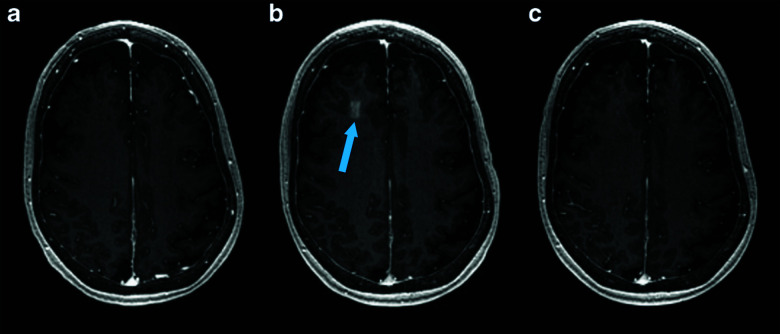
MRI images from baseline (a), immediately following HIFU (b), and 24 hours after HIFU (c), demonstrating opening and closure of BBB. Extravasation and pooling of contrast within the insonated portion of the frontal lobe is visible immediately following HIFU administration (b, blue arrow) demonstrating opening of the BBB, but is absent 24 hours later (c) suggesting BBB closure. Reprinted (modified) with permission from ref. 116.
